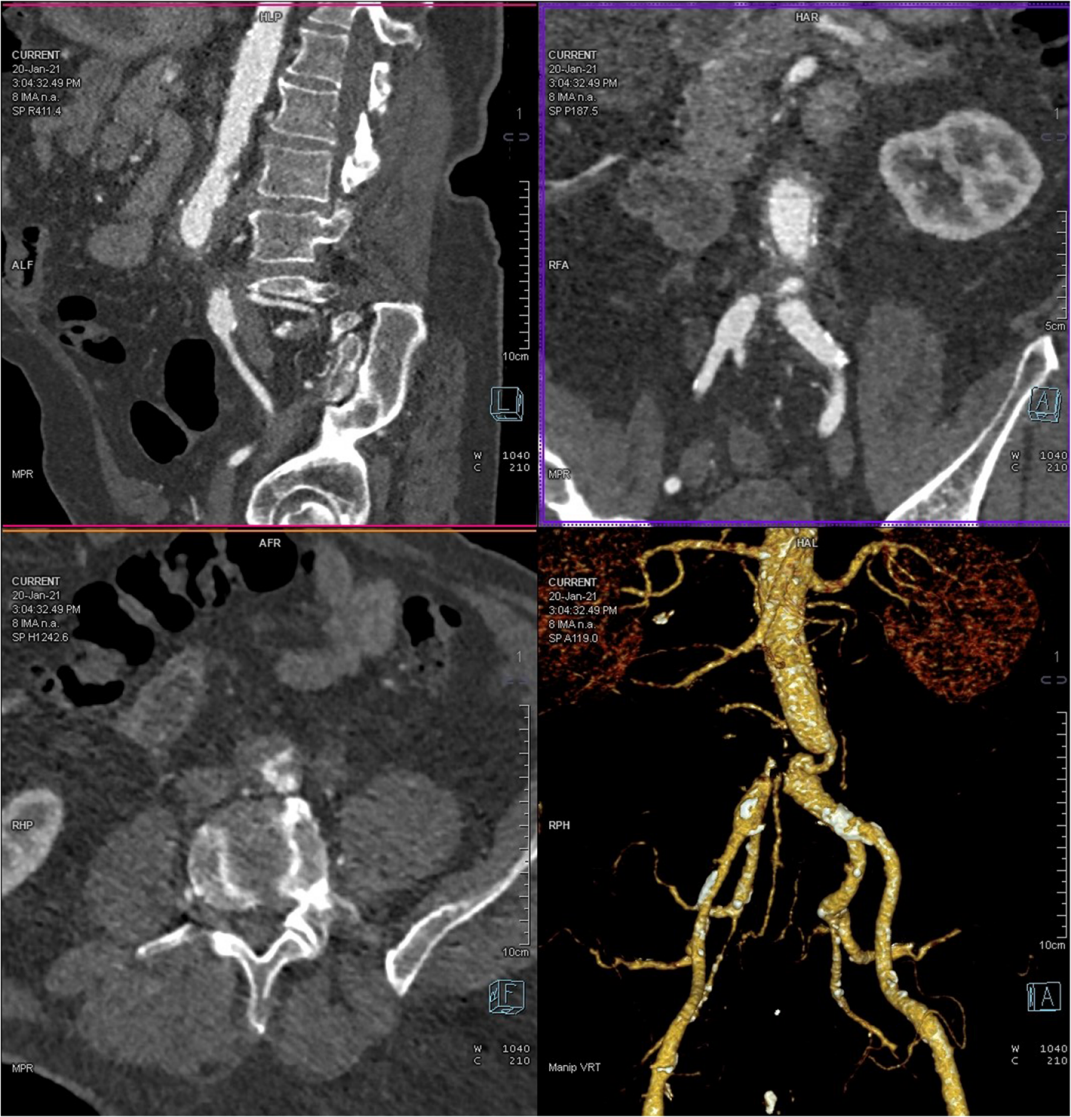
a CT angiography images performed before the procedure
